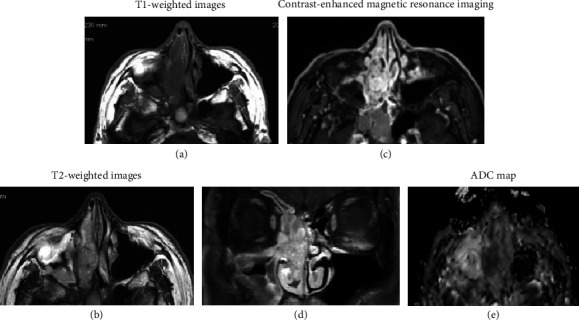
Magnetic resonance imaging findings. Axial (a) T1-weighted and (b) T2-weighted images show a mass with low signal intensity on T1 and mildly high signal intensity on T2 in the right paranasal sinus. (c) Contrast-enhanced T1-weighted image shows strong enhancement of the lesion. (d) Coronal Contrast-enhanced T1-weighted image demonstrates a hyperintense signal surrounding the mass. (e) Diffusion-weighted image shows high signal intensity in the periphery of the lesion, with a corresponding low apparent diffusion coefficient value, suggesting malignancy.
